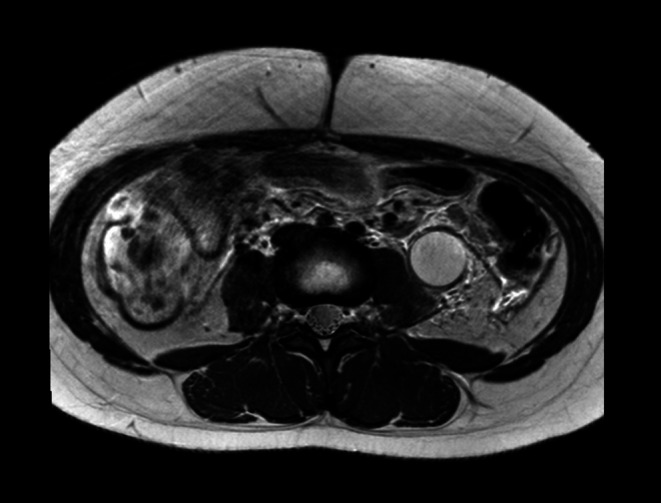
Multiparametric pelvic MRI, axial T2W TSE HR sequence showing ectatic left megaureter with diffusely overfluid contents and concentrically thickened and hyperintense walls.
